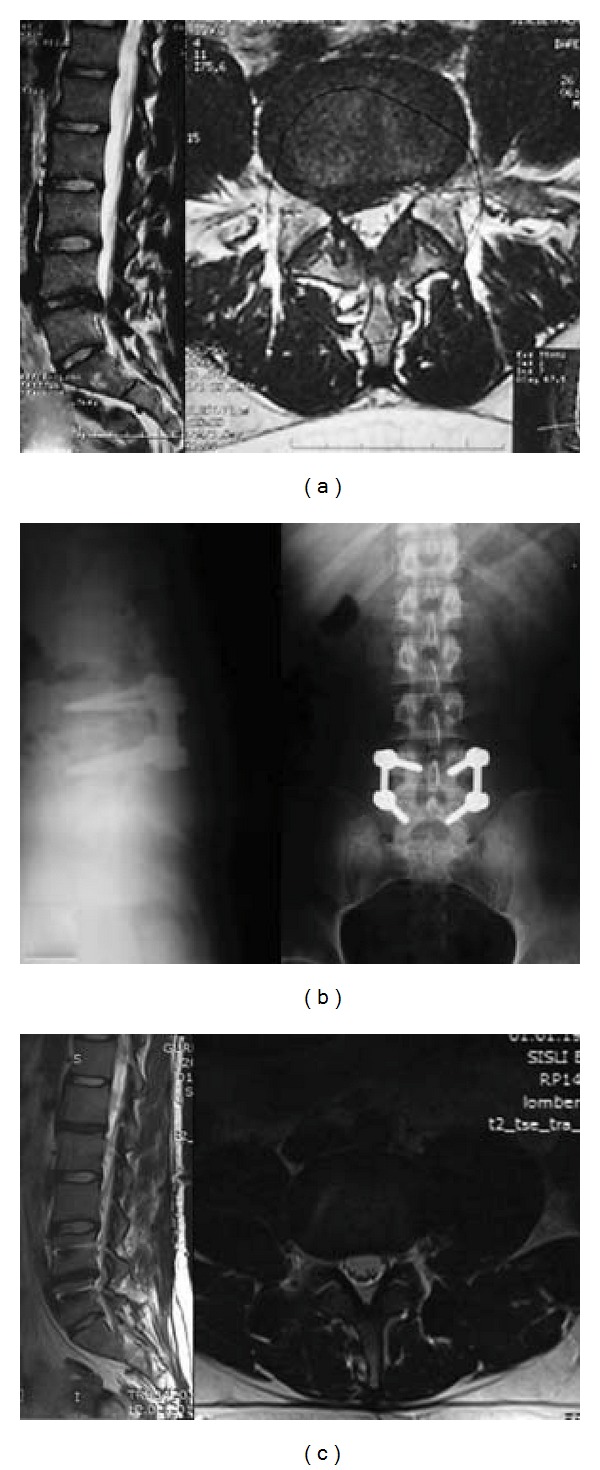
18-year-old, male patient with severe acute back pain attacks. In the last attack, he became aware of sciatalgia on the right side. He had no response to medical treatment and physical therapy; he had difficulties in daily life. (a) Preoperative MRI showed posterior annular defects, and a bulging disc to the right L5 nerve root is compressed; (b) saphinas system was applied; (c) one year after surgery the patient is very satisfied with this surgery, and MRI showed no bulging or defects of posterior annulus.
